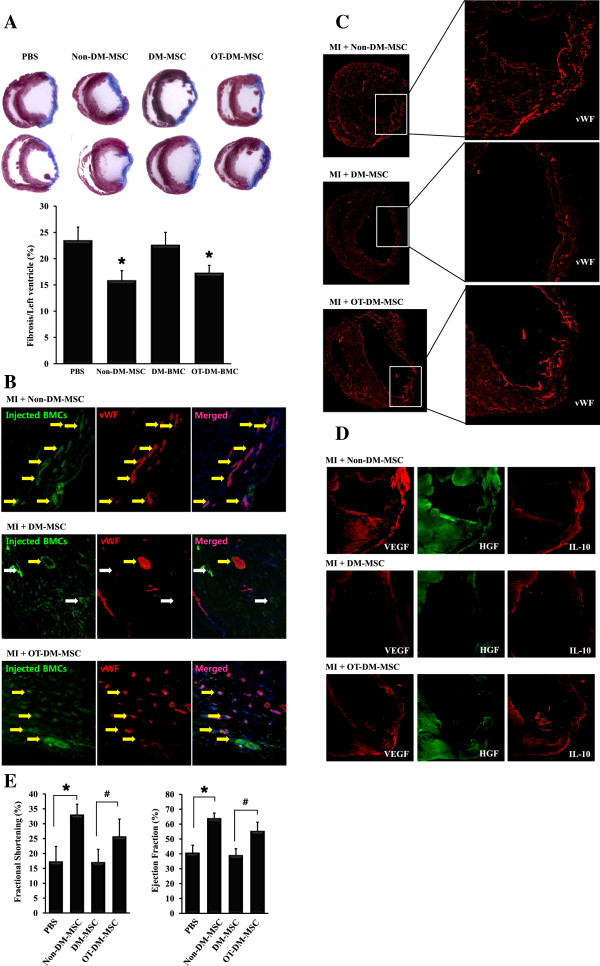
Cardiac fibrosis, expressions of angiogenic factors, and cardiac function were ameliorated by injection of OT-DM-MSC in infarcted myocardium. (A) Representative images showed fibrosis was significantly reduced in Non-DM-MSC and DM-MSC-injected heart. (B) Representative images of GFP-labeled MSCs (green) injected in infarcted myocardium showed expression of von Willebrand factor (vWF, red). The merged images showed vWF-expressing MSC (yellow arrows) and none-expressing MSC (white arrows). (C) Distribution and degree of vWF expression in the whole heart. Less vWF and restored vWF were observed in DM-MSC injected heart and DM-MSC injected heart after MI, respectively. (D) Expressions of vascular endothelial growth factor (VEGF), hepatocyte growth factor (HGF), and interleukin-10 (IL-10) in infarcted myocardium were examined. (E) Echocardiographic evaluations were performed 2 weeks after cell injection. * *p* < 0.01 vs PBS; # *p* < 0.01 vs DM-MSC.
